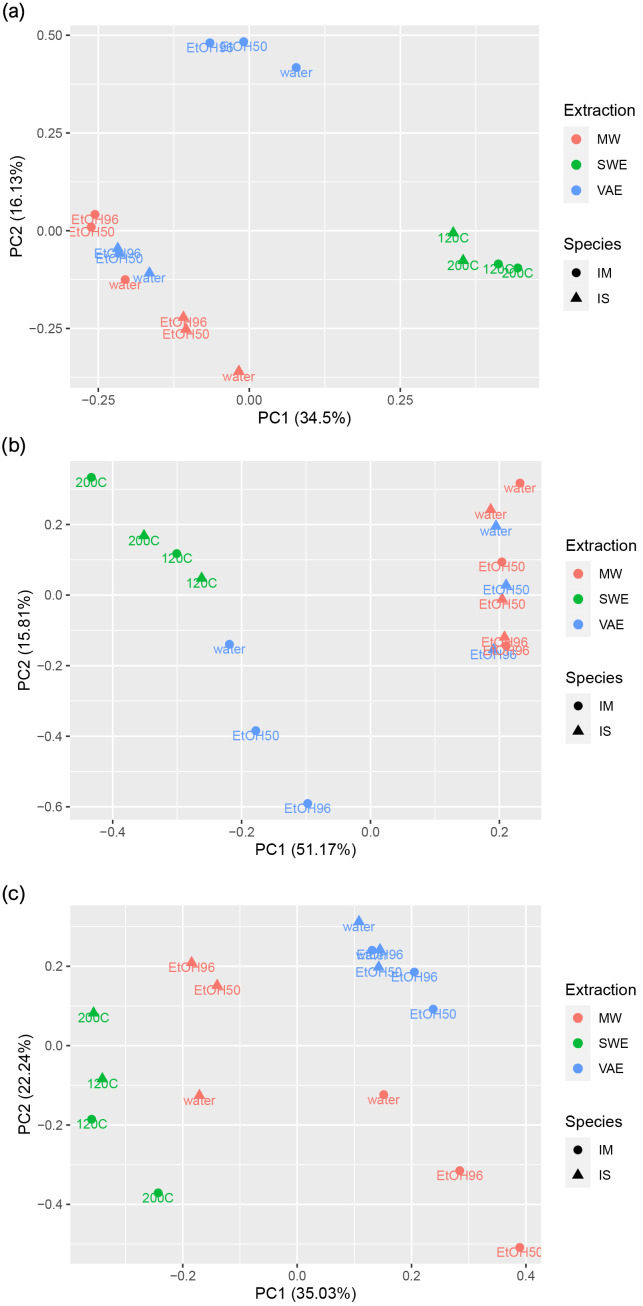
Principal component analysis for (a) the yield and total 51 examined compounds (b) TPC and phenolic compounds (c) TPS and sugar content; IS—Serbian Chaga, IM—Mongolian Chaga, MW 96% EtOH, 50% EtOH, H_2_O—microwave-assisted extraction, VAE 96% EtOH, 50% EtOH, H_2_O—ultrasound-assisted extraction, SWE 200 °C, 120 °C—subcritical water extraction. IM (point) and IS (triangle); TPCs, total phenolic compounds; TPSs, total polysaccharides; Different extraction techniques are expressed in different colors, while the names below point/triangle indicate the solvent/conditions used.
